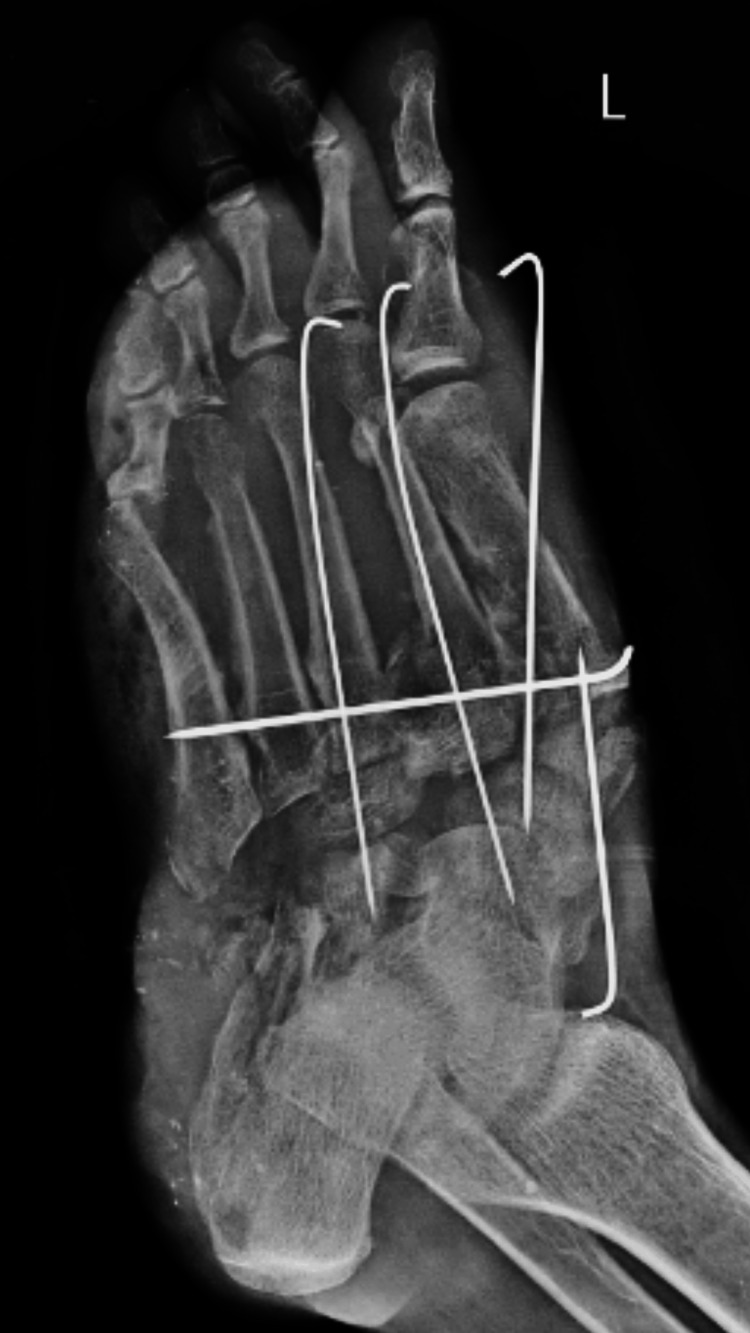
Postoperative X-ray of the ankle and toes X-ray shows the K-wire fixation of the cuneiform and cuboid fracture and horizontal K-wire fixation for the stability of the left ankle K-wire: Kirschner wire
